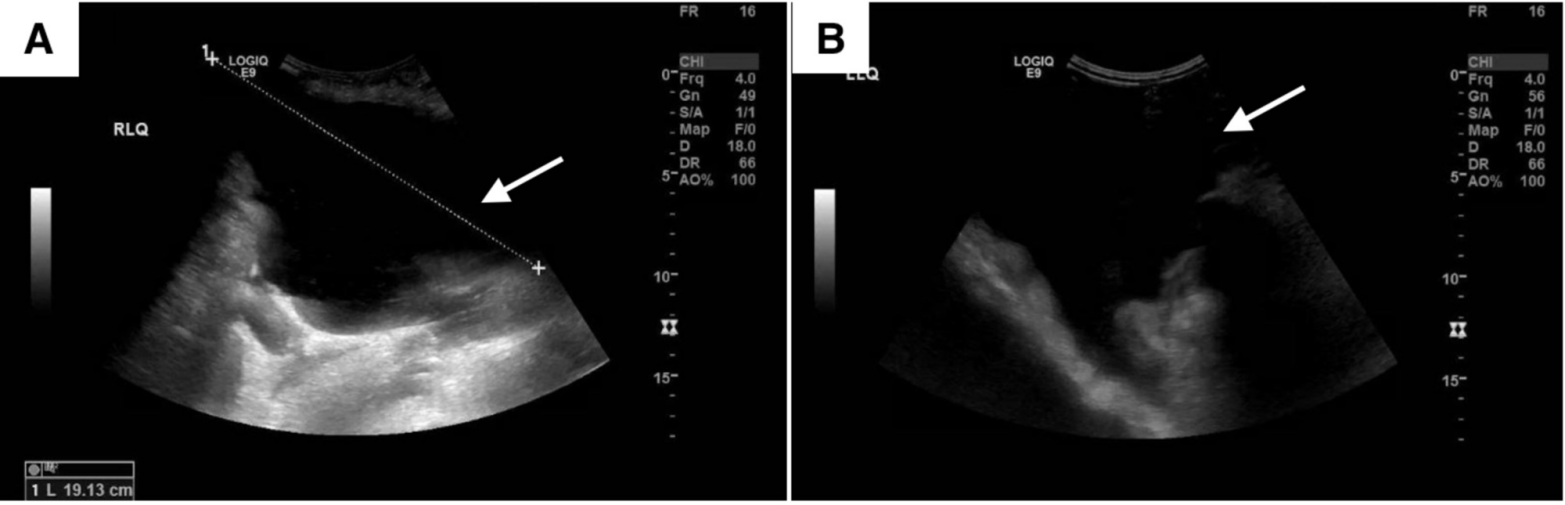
Sonographic evidence of large abdominopelvic ascites of the right lower quadrant (A) and left lower quadrant (B). The largest pocket is 19.13 cm in the right lower quadrant.
